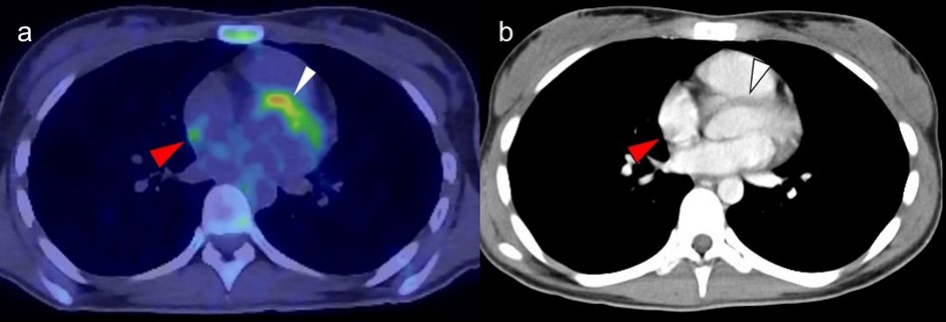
Crista terminalis. Mild focal FDG uptake was observed in the right atrium (arrowhead) (a), which corresponded to the crista terminalis confirmed by contrast enhanced CT (b). In addition, linear FDG uptake correspond to the fat tissue suggested lipomatous hypertrophy (white arrowhead)
